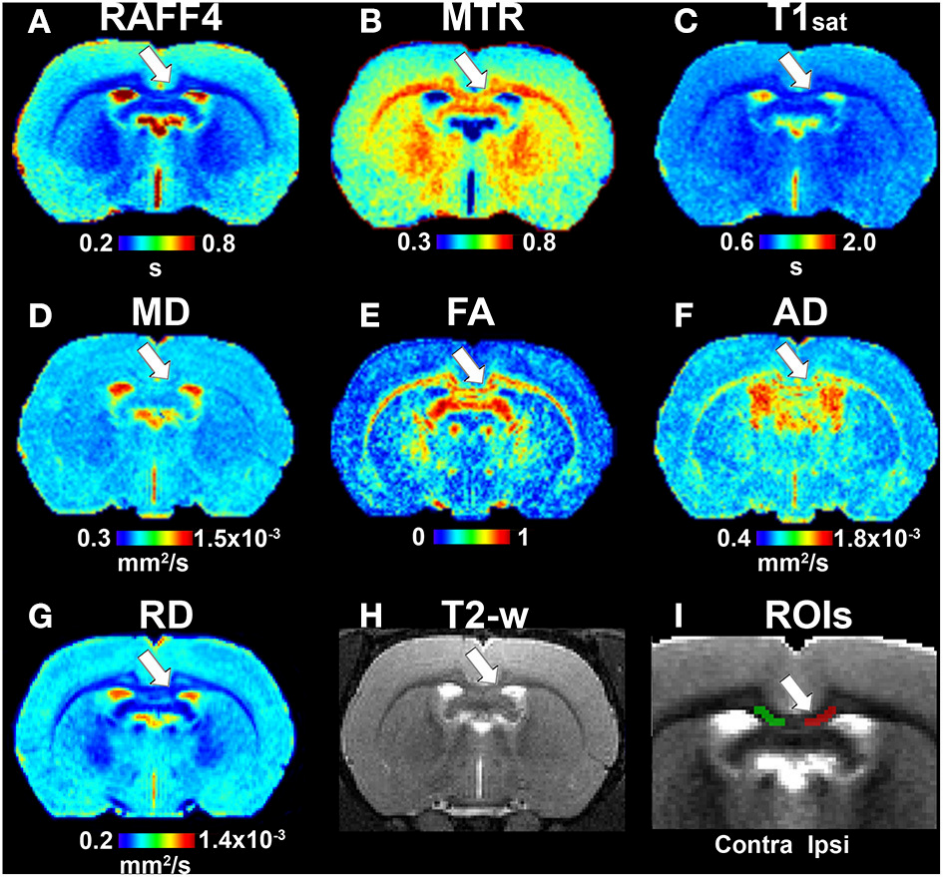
Quantitative MRI maps in the remyelination phase, on day 38. Relaxation time constant map of RAFF4 (A), magnetization transfer ratio, MTR (B), T1sat (C), mean diffusivity, MD (D), fractional anisotropy, FA (E), axial diffusivity, AD (F), radial diffusivity, RD (G), T2w image with the lesion (H) and a representative example of ROIs for analyzing lesion on a grayscale RAFF4 map (I). White arrow points to the lesion in the corpus callosum.
